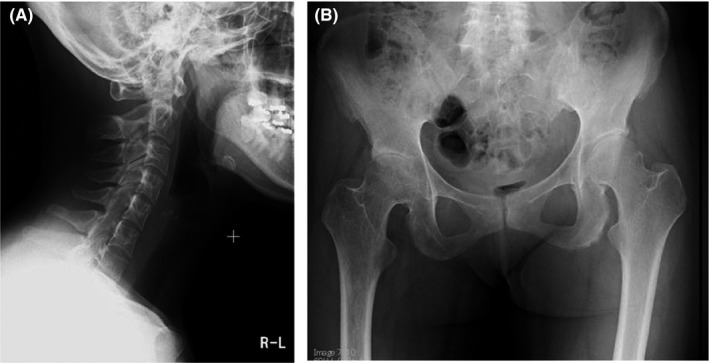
Radiograph of the patient at 33 y of age when ankylosing spondylitis was diagnosed. A, Radiograph of the neck. Osteosclerosis of the posterior surface of cervical vertebra was observed. B, Radiograph of both hips and the pelvis. Osteoarthritis of the right hip with joint space narrowing was observed
